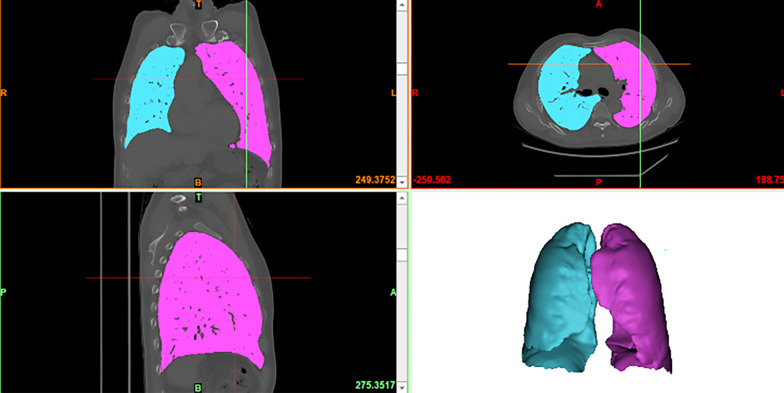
Coronal, axial and sagittal CT images and the TLV (CT threshold, − 1024 HU to − 500 HU)
